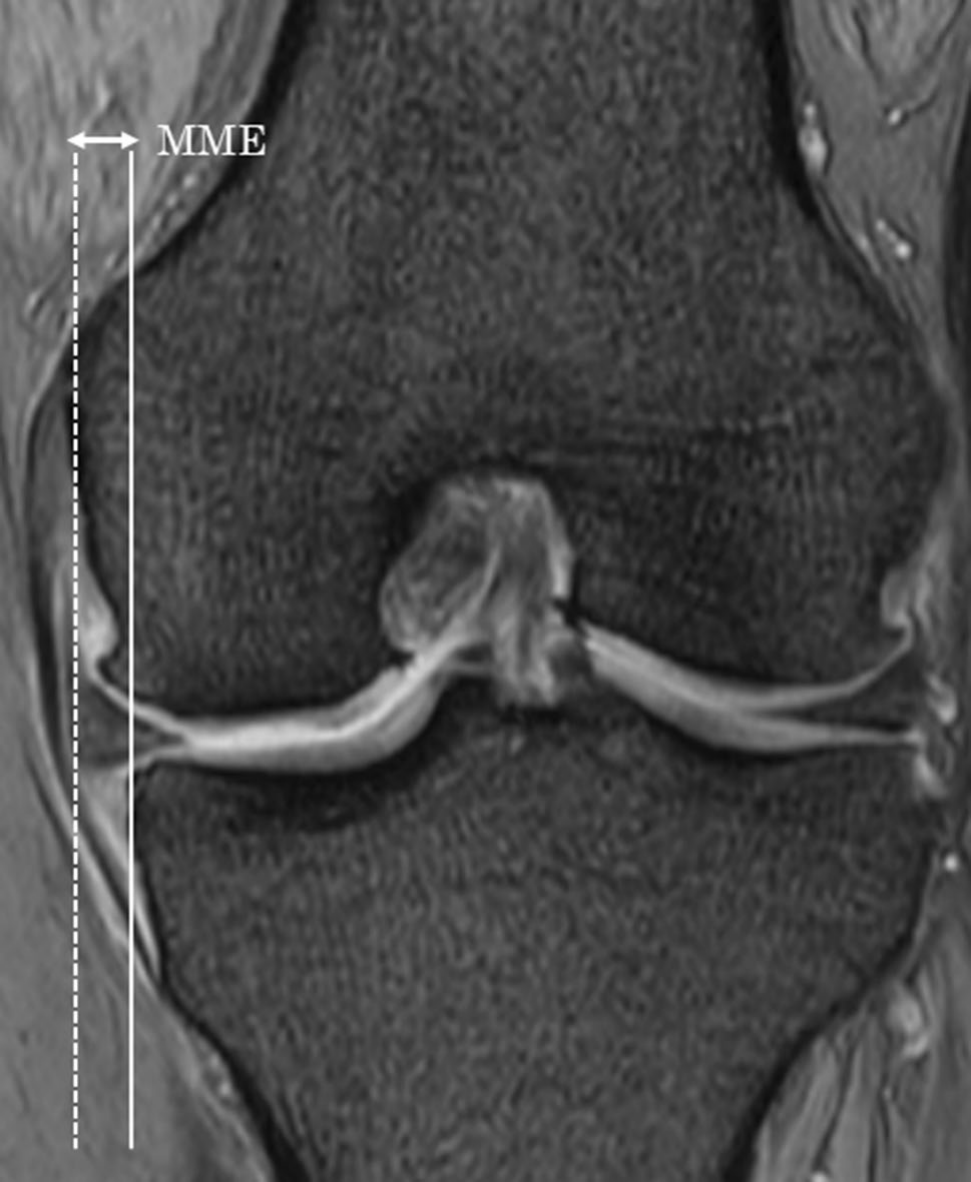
Methods for evaluating MME via coronal plane MRI. *MME* medial meniscal extrusion, *MRI* magnetic resonance imaging.
